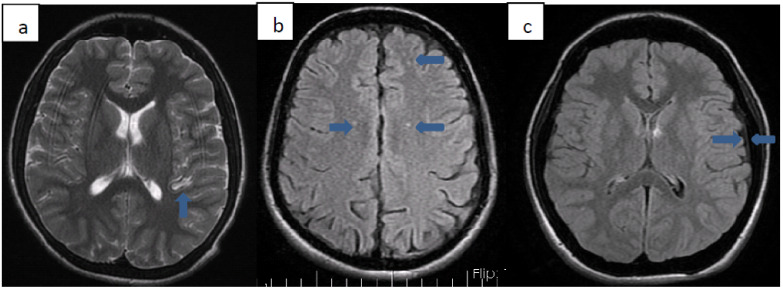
(a–c): Axial T2 (a) and Axial FLAIR (b) weighted images show multiple foci of hyperintensity that are related to asymptomatic brain ischemia. Axial FLAIR (c) image show widened epidural tissue.
